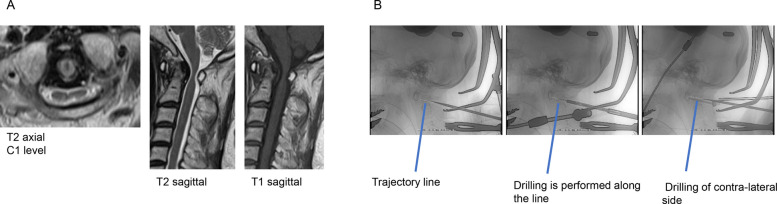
Presentation of case 2. A Pre-operation magnetic resonance imaging images. B Insertion of screws along the virtual insertion line
